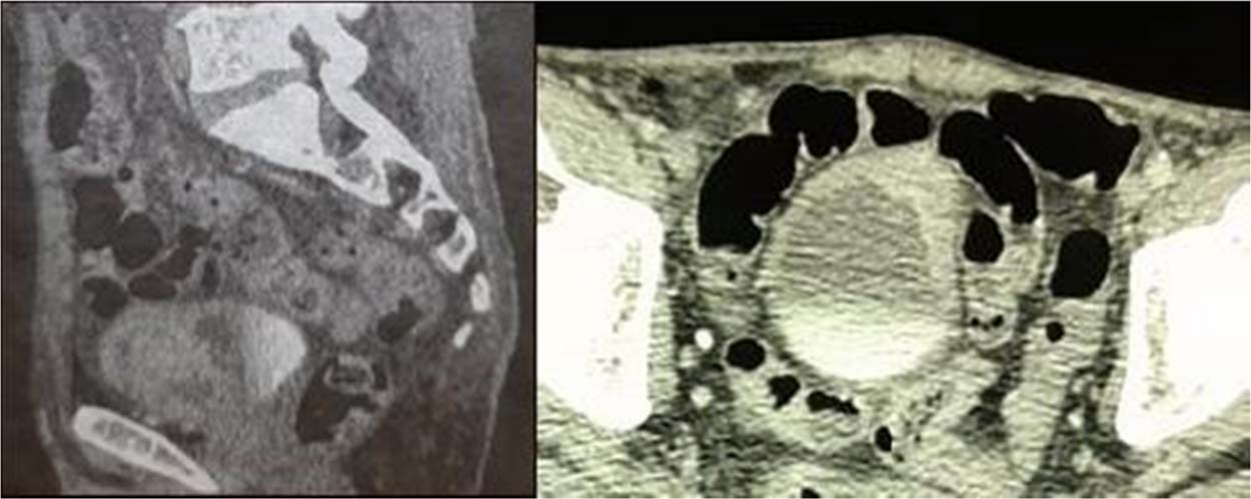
Computed tomography scan showing an enhancing, pedunculated lesion arising from the anterior and left lateral bladder wall (plasmacytoid urothelial carcinoma of the bladder).
